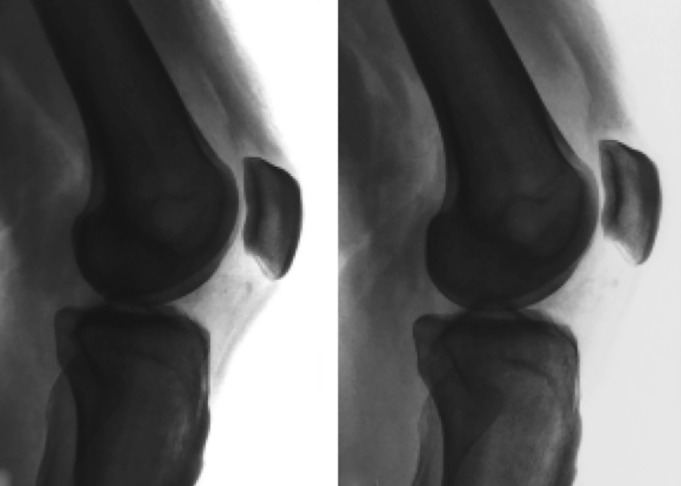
Effect of weight bearing (WB) (image on the right) on patellar proximal displacement in lateral radiographs^7^.
